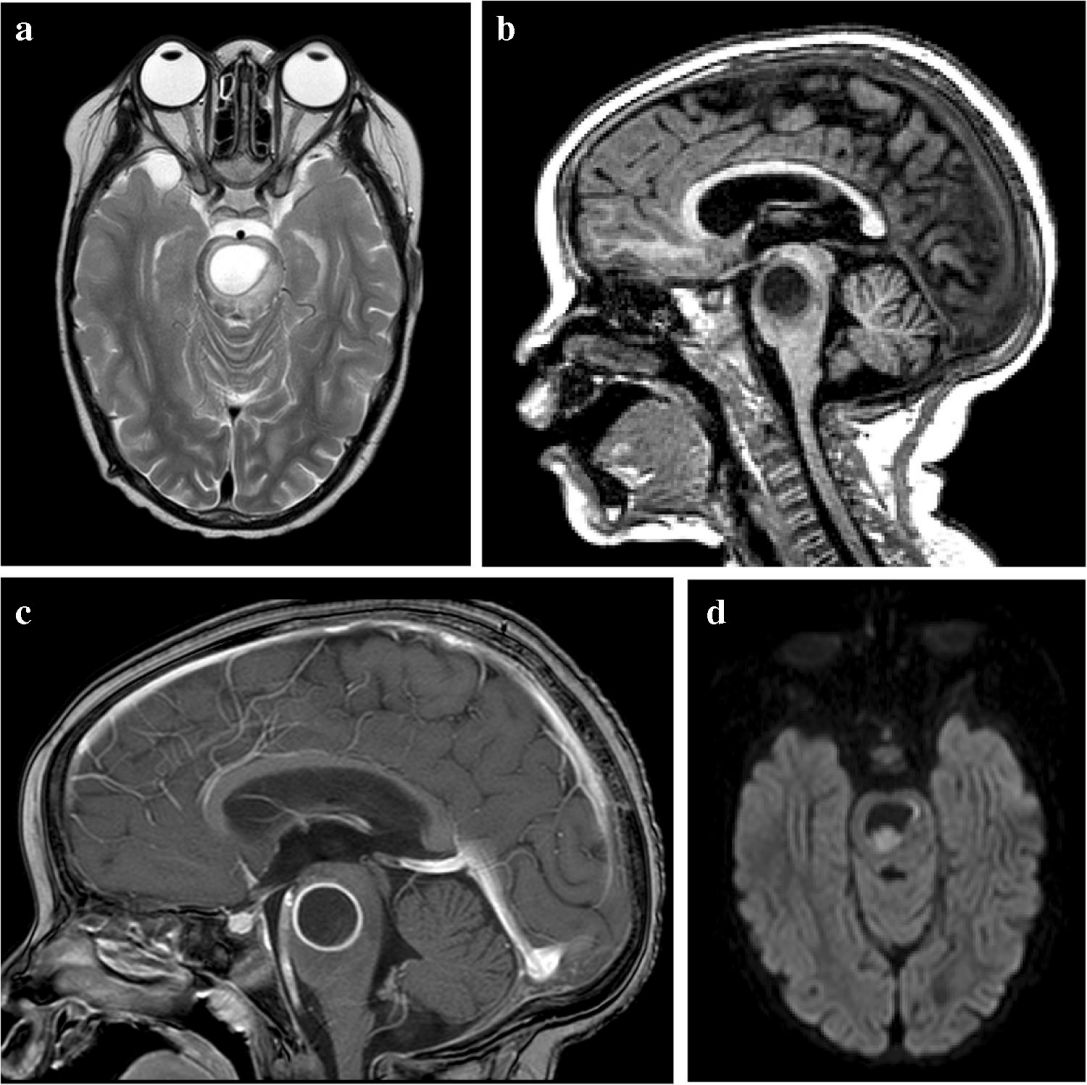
Brain magnetic resonance imaging in a 12-year-old boy a Axial T2 demonstrates a hyperintense lesion in the pons with a hypointense rim. b, c Sagittal images show intermediate to low signal on T1 (b) with peripheral enhancement post-gadolinium (c). d Axial diffusion weighted imaging shows some restriction in the wall and content. There was corresponding low signal on apparent diffusion coefficient map (not shown). The differential for these imaging findings includes a tuberculous (TB) abscess or liquifying tuberculoma. The patient’s clinical condition deteriorated requiring drainage; surgery confirmed a TB abscess with the content teeming with TB bacilli
